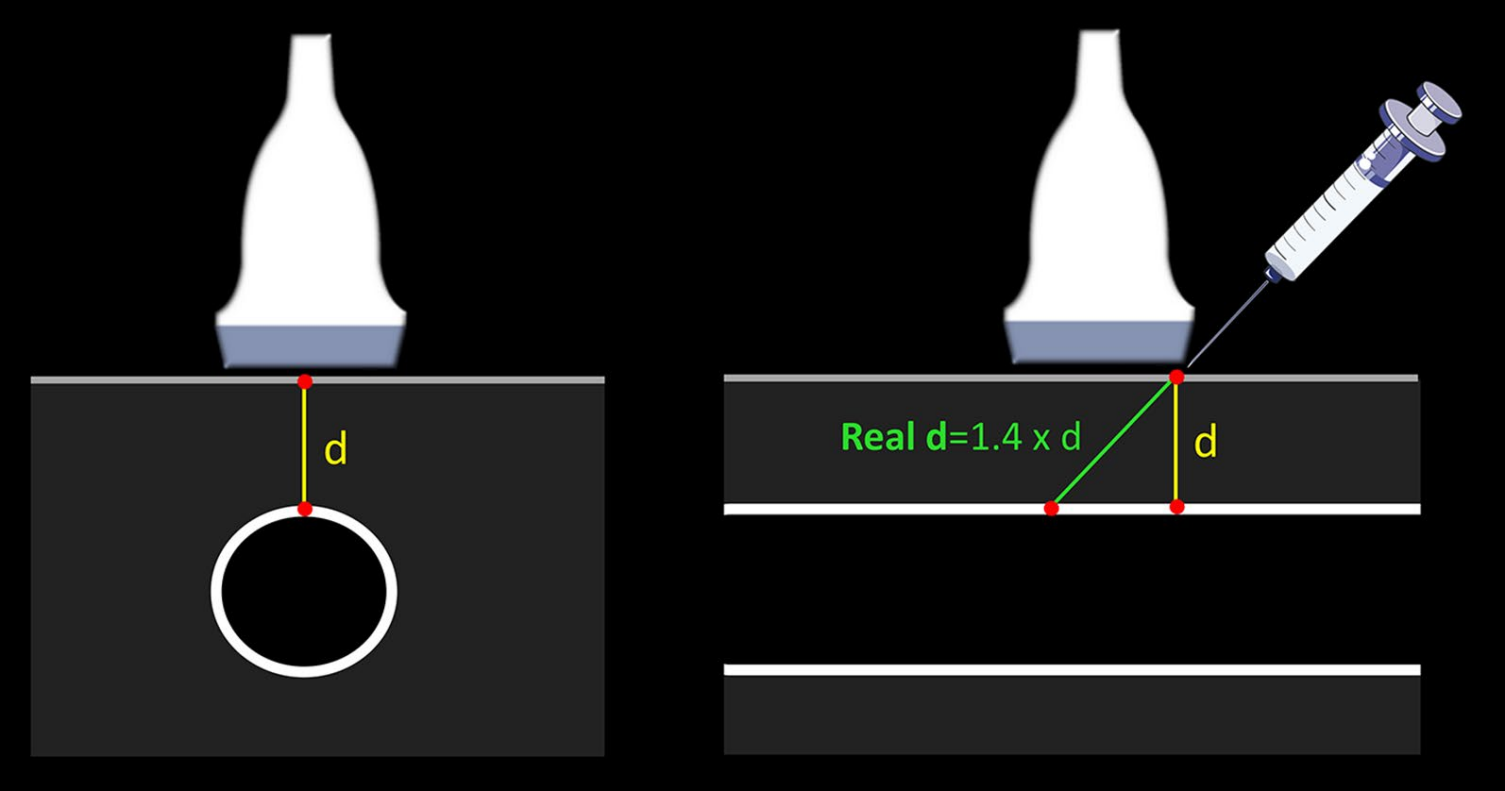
Explaining the distance from skin to vessel in ultrasound-guided cannulation. While this distance (*d*) is estimated in the short axis, the real distance to reach the vein depends on the insertion angle. Assuming a 45° insertion angle, this real distance is equal to *d* multiplied by 1.4. Of note, the real distance decreases with sloped insertions, and increases using shallower insertions
